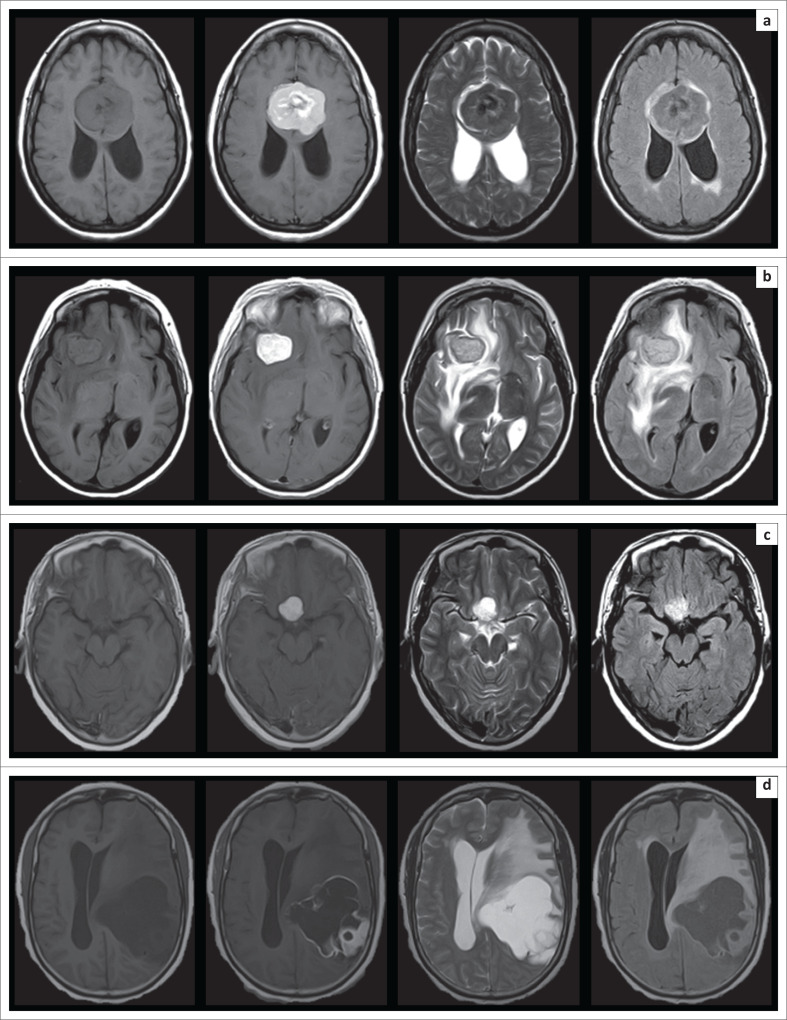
Selected axial MRI brain images depicting the appearances of the different World Health Organization (WHO) Grade I uncommon meningioma subtypes encountered: (a) Psammomatous meningioma, (b) Secretory meningioma, (c) Microcystic meningioma and (d) Mixed angiomatoid-microcystic meningioma (shown from left to right on T1-weighted imaging [T1-WI], T1-WI post-gadolinium, T2-weighted imaging [T2-WI] and fluid attenuation inversion recovery [FLAIR] sequences).
